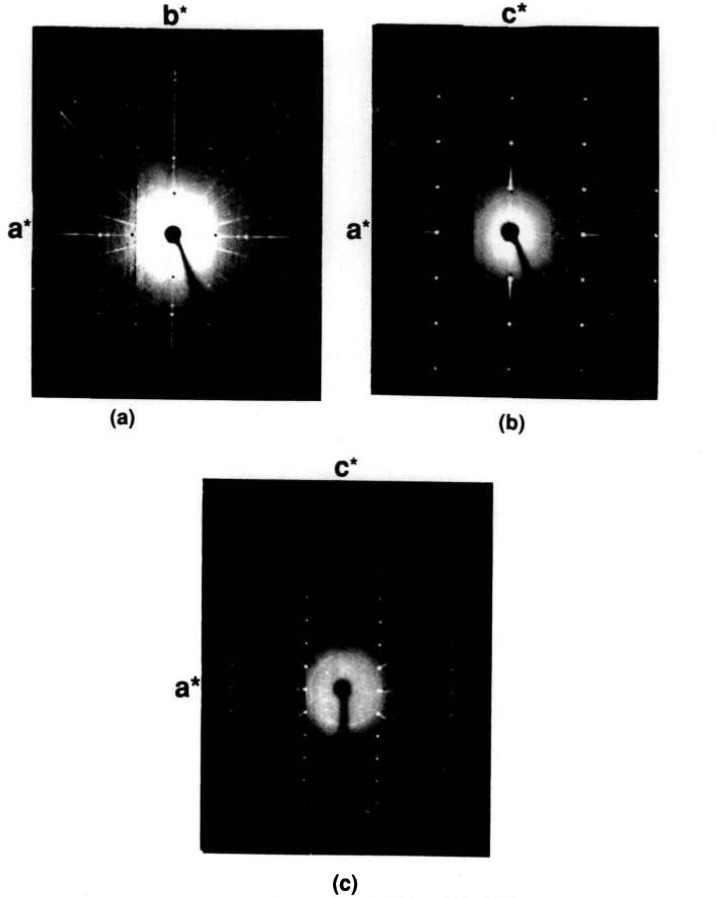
X-ray precession photographs of Sr_2_Bi_2_O_5_ (a) *hk0*, (b) *h0l* and (c) *hIl.*
